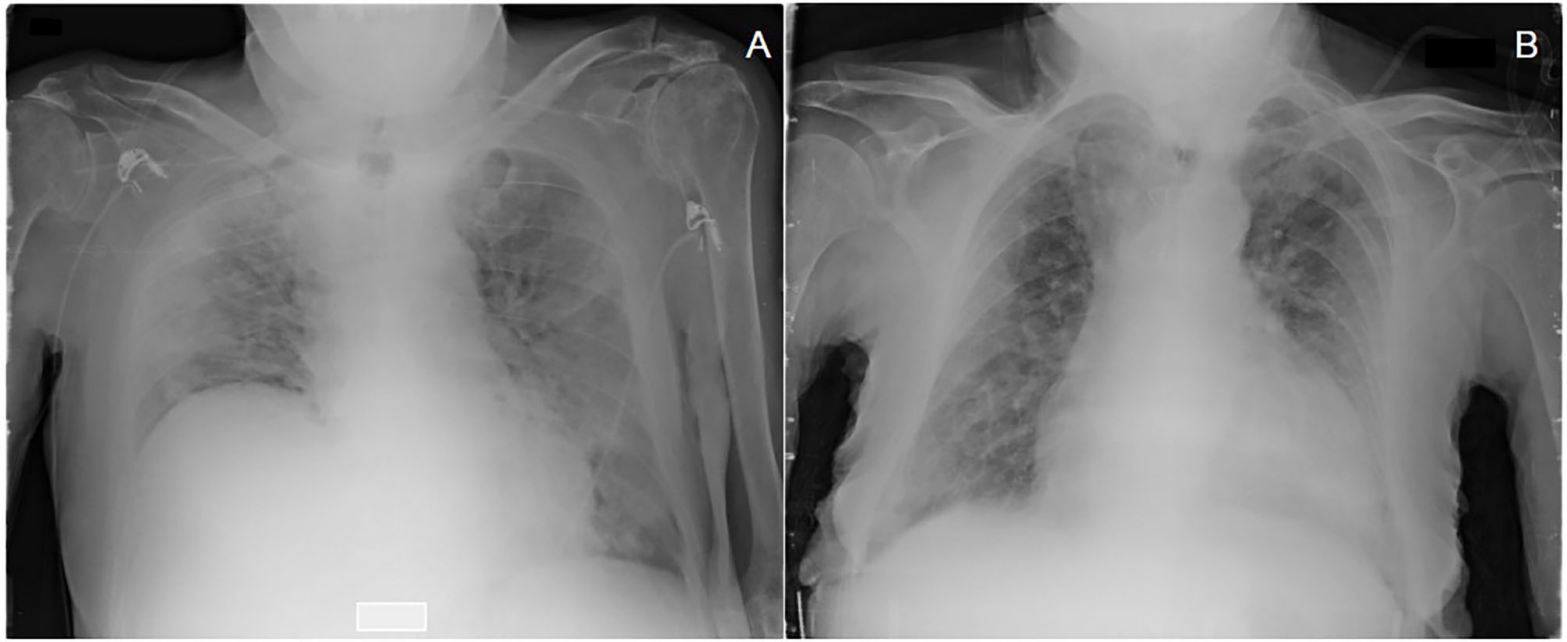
Chest X-ray with a high CARE score at admission of two patients treated with high intensity medical care (HIMC) during hospitalization and with different values of viral load (Gene E) at diagnosis: (A) a 81 years old patient presenting a CARE score of 22 points and a viral load of 2.43 log10 copies/ml (<25th percentile); (B) a 92 years old patient presenting a CARE score of 18 points and a viral load of 6.72 log10 copies/ml (>75th percentile).
